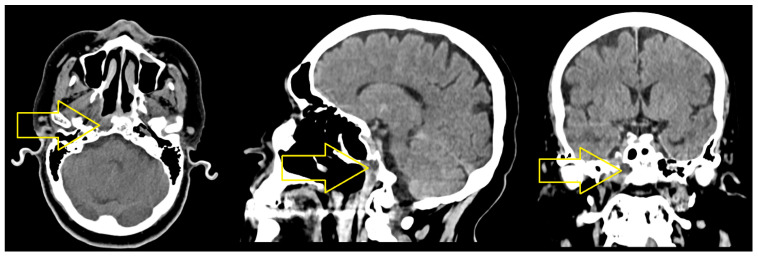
The CT scan of the cervical area with contrast medium—October 2021. Moderate diffuse thickening of the mucosa of the cavity of 5 mm (yellow arrow).
